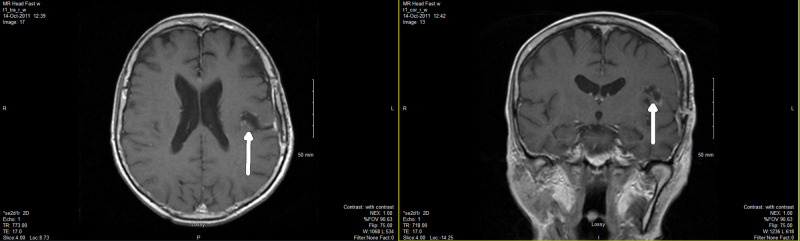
Post-WBI MRI showing no residual disease in the surgical bed or other part of the brain WBI, whole brain irradiation
